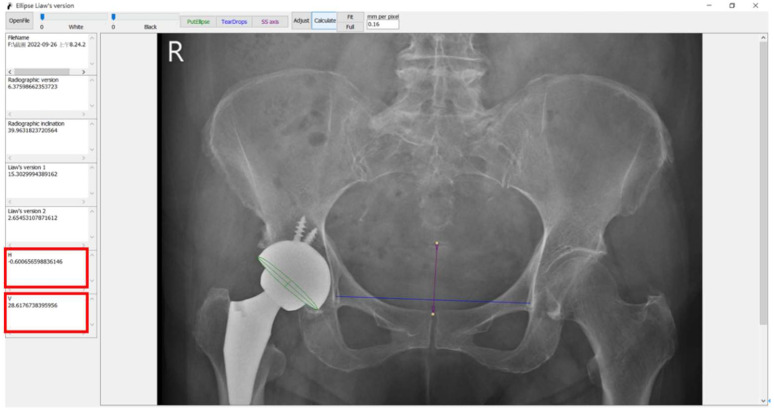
The application of the software to measure pelvic rotation. The purple line represents the radiographic pelvic axis (SCSP). The blue line represents the trans-teardrop line (TTDL). The green oval line represents the edge of the acetabular component of THA. The absolute value of H in Figure 2 was h. By applying the angle of intersection of TTDL and SCSP, we could calculate the value of ssd.
